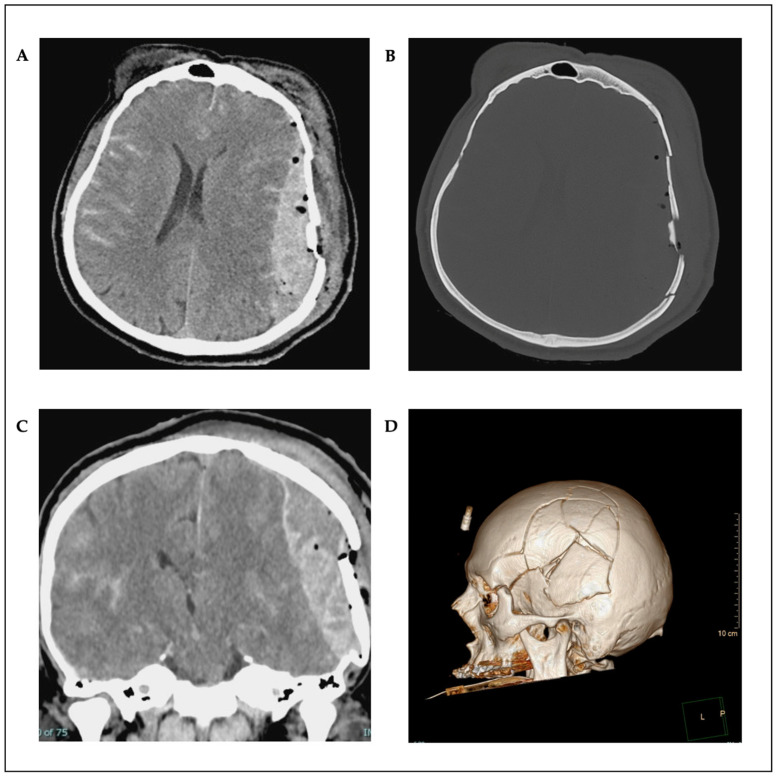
Preoperative axial (A,B) and coronal (C) CT scans; 3D reconstruction (D).
